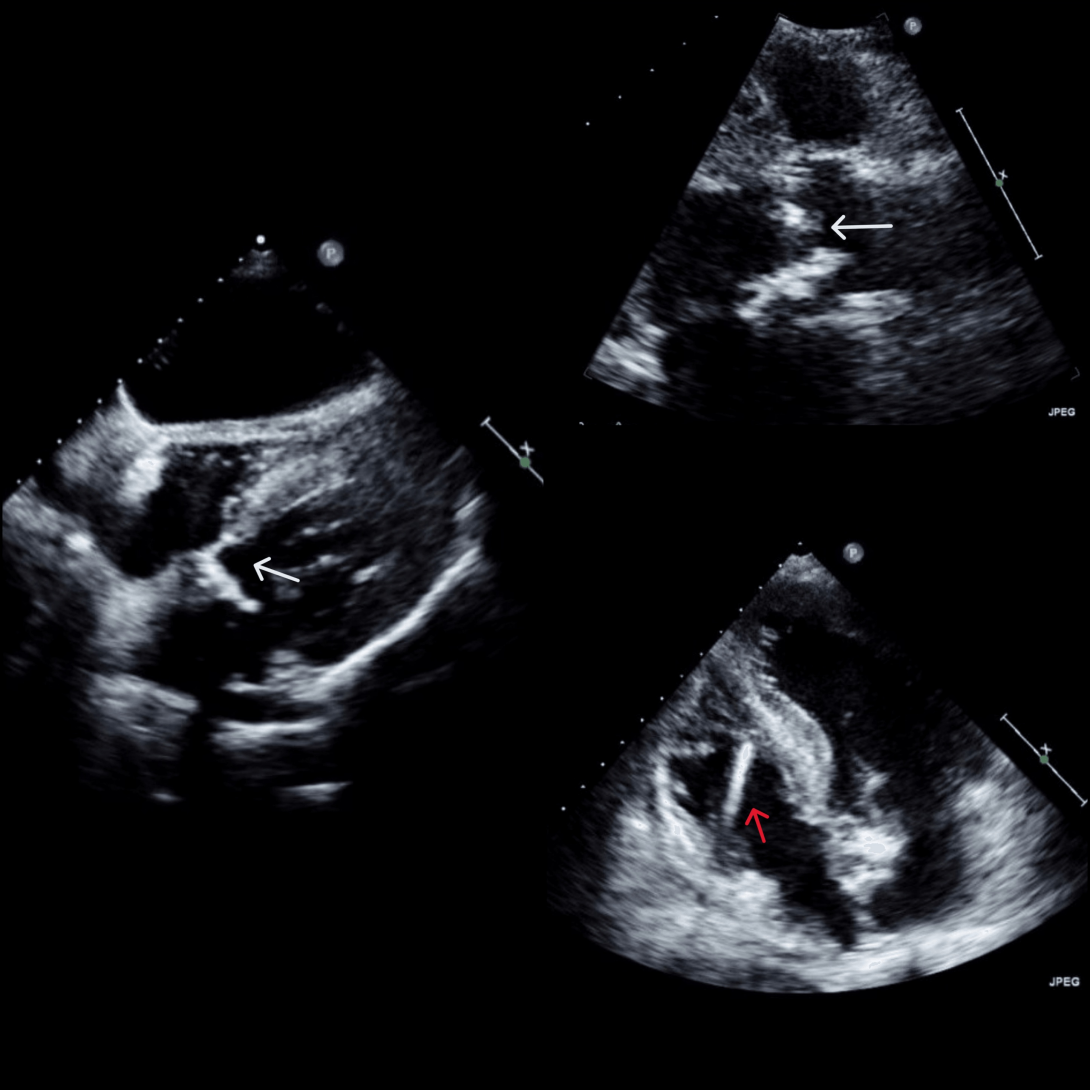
TTE showing aortic valve vegetations as mobile echogenic structures (white arrows), visible in the parasternal long-axis view (left and upper right images). Additionally, the pacemaker wire (red arrow) is seen in the right heart chambers without vegetations, as observed in the apical four-chamber view (bottom right image) TTE: transthoracic echocardiogram
